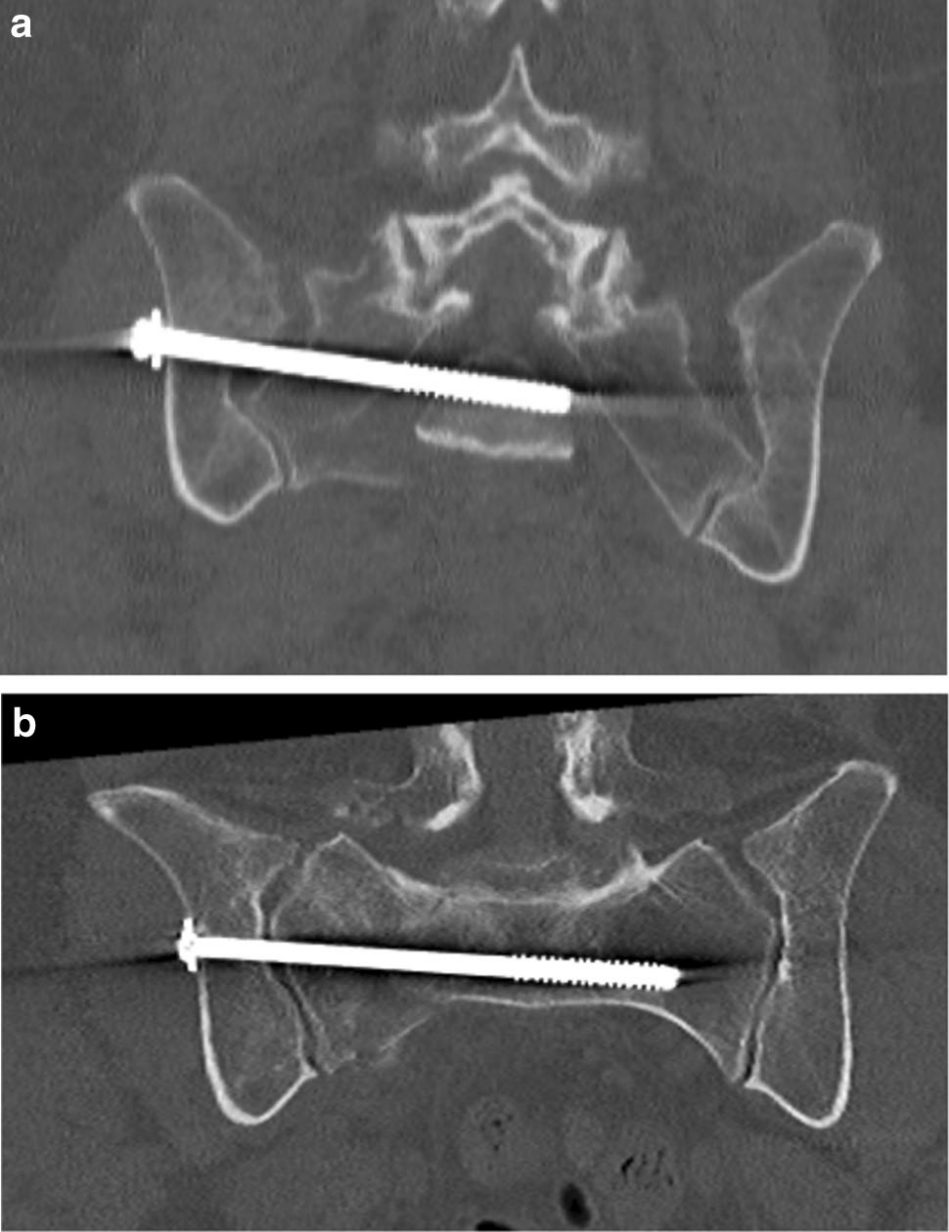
a Postoperative CT-scan in a 60-year female with a vertical sheer type fracture displaying an SI-screw penetrating the right and touching the left S1 foramina. b Postoperative CT-scan in the same patient after reoperation with exchange of the SI-screw
